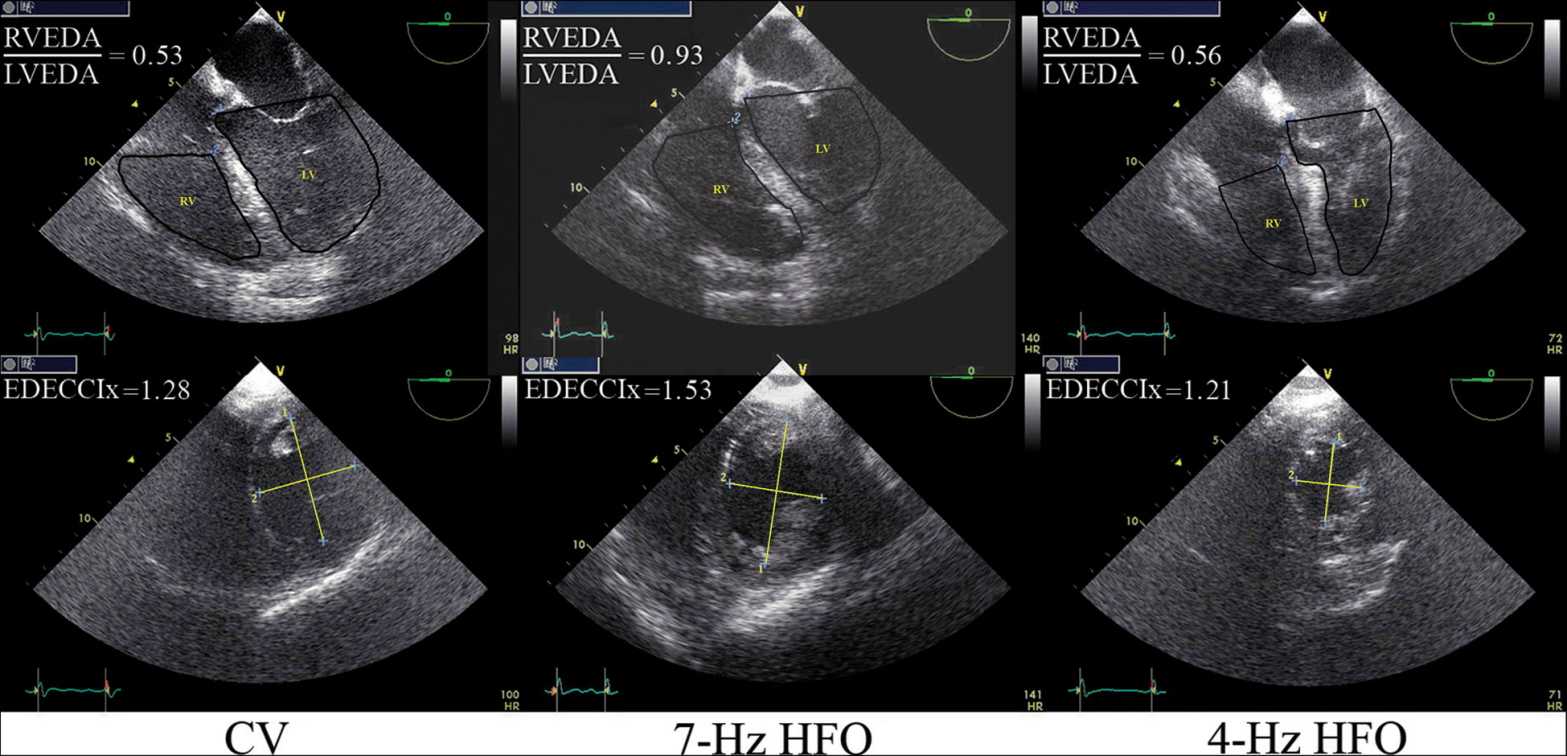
Examples of transesophageal echocardiographic (TEE) determination of two primary study outcome variables. Upper panel: determination of the right-to-left ventricular end-diastolic area ratio (RVEDA/LVEDA). Lower panel: determination of the end-diastolic eccentricity index (EDECCIx). The left vertical pair of images was obtained from study participant no. 12 during conventional ventilation (CV), the middle pair of images was obtained from participant no. 7 during 7-Hz high-frequency oscillation (HFO), and the right pair of images was obtained from participant no. 13 during 4-Hz HFO. In the lower panel (short-axis, transgastric views), “2” corresponds to the diameter of the left ventricle (LV) that was perpendicular to and bisecting the interventricular septum, whereas “1” corresponds to the diameter that was perpendicular to “2.” TEE measurements were repeated and averaged over 2–4 consecutive cardiac cycles (see also Additional file 1); EDECCIx was calculated as diameter “1”/diameter “2.” Description of figure modifications performed with Photoshop CC (Adobe Systems): upper panel, enhancement of the periphery of the right ventricle (RV) and LV using the “pen” tool and the “stroke path” command and enhancement of contrast of the middle image using the “adjust levels” command; lower panel, enhancement of diameter “1”/diameter “2” using the “line” tool. The original versions of the stored pairs of images are also provided in Additional file 1: Figure S1
